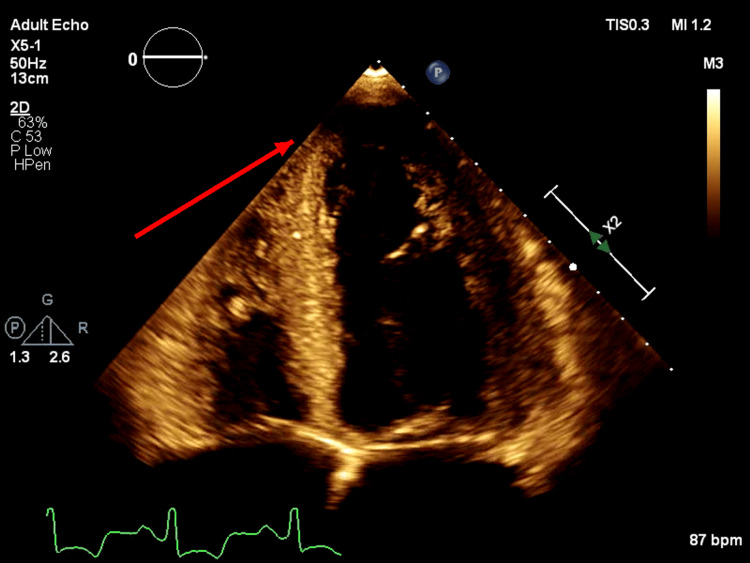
Repeat transthoracic echocardiography demonstrating the interval resolution of the left ventricular apical thrombus with prominent apical trabeculations
